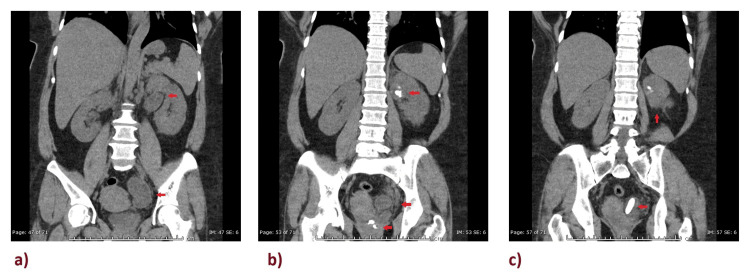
Selected coronal images from the CT urinary tract showing tortuous dilatation of the left ureter Image A reveals hydroureteronephrosis confined to the upper pole with a dilated pelvicalyceal system (red arrows). Image B shows multiple obstructive renal and ureteric calculi with significant periureteric fat stranding (red arrows). Image C points out a large obstructive distal ureteric calculus with associated left perirenal fat stranding (red arrow).
